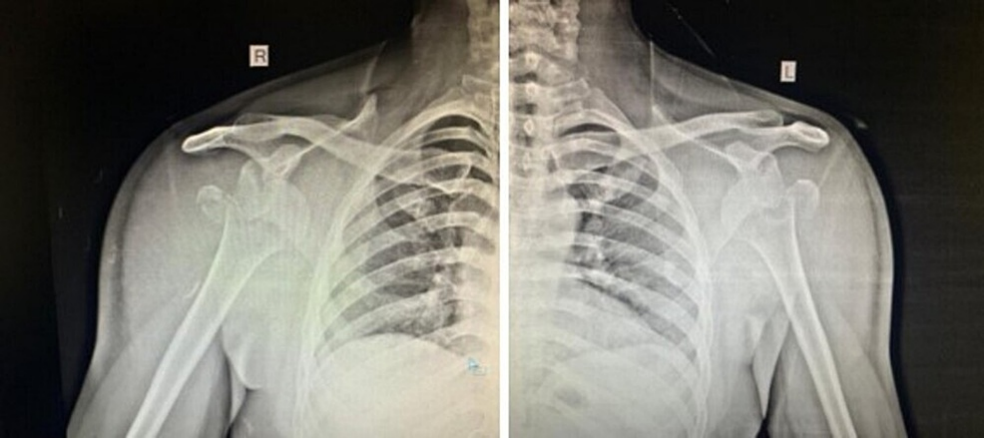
Pre-reduction radiographs showing bilateral shoulder dislocation with displaced greater tuberosity fractures
